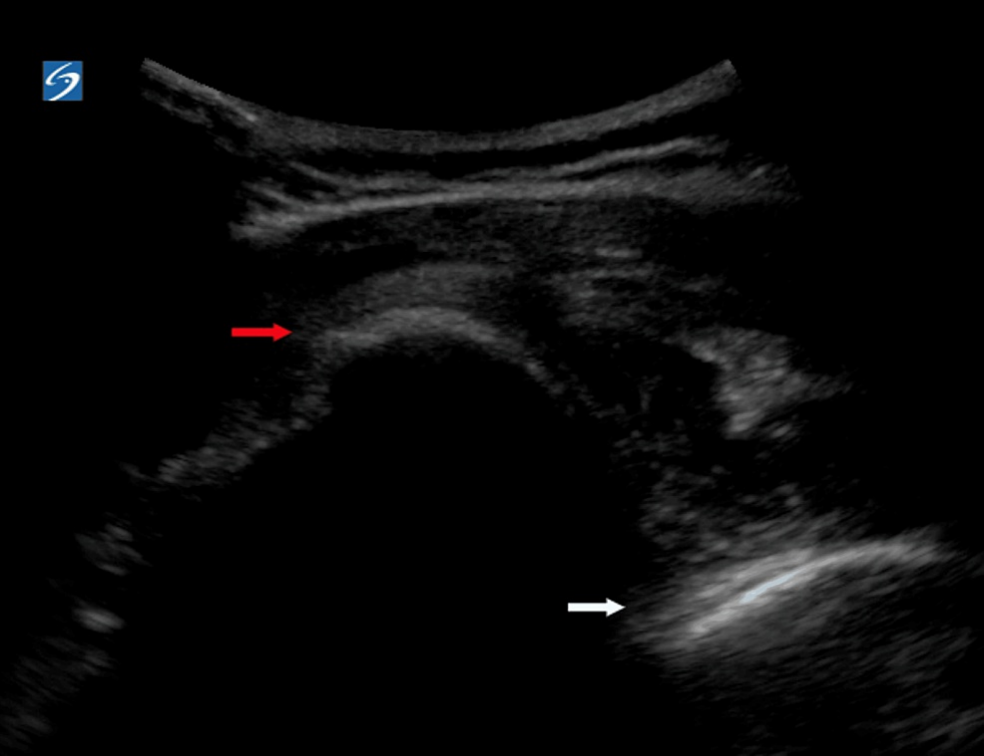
Pre-reduction point-of-care ultrasound of the left shoulder using a curvilinear probe and a posterior approach demonstrating posterior displacement of the humeral head (red arrow) relative to the glenoid rim (white arrow)
